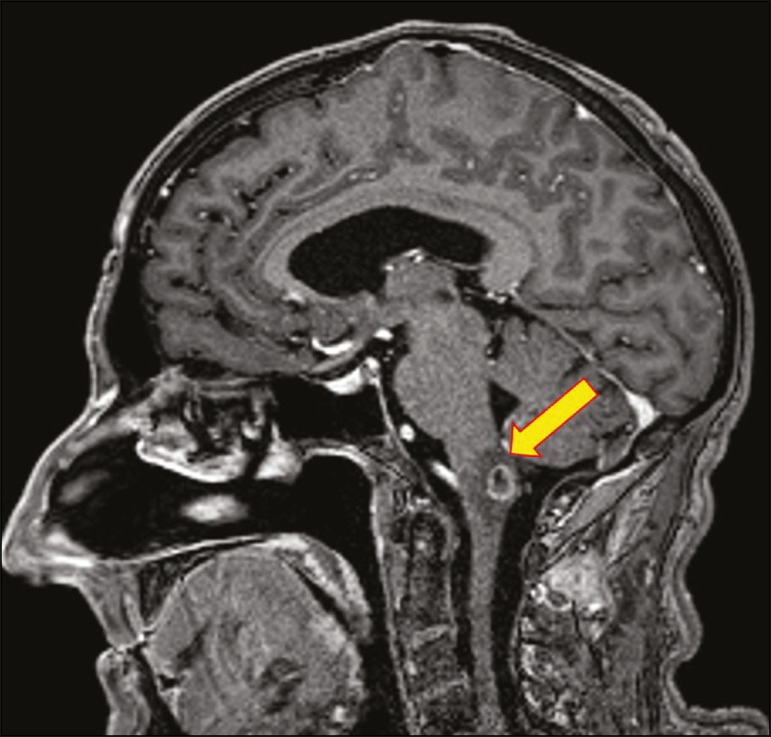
Cryptococcomas caused by *Cryptococcus gattii*. Gadolinium contrastenhanced sagittal T1-weighted MRI sequence showing a hypointense lesion with peripheral ring enhancement in the bulb (arrow), with signs of edema in the surrounding tissues.
